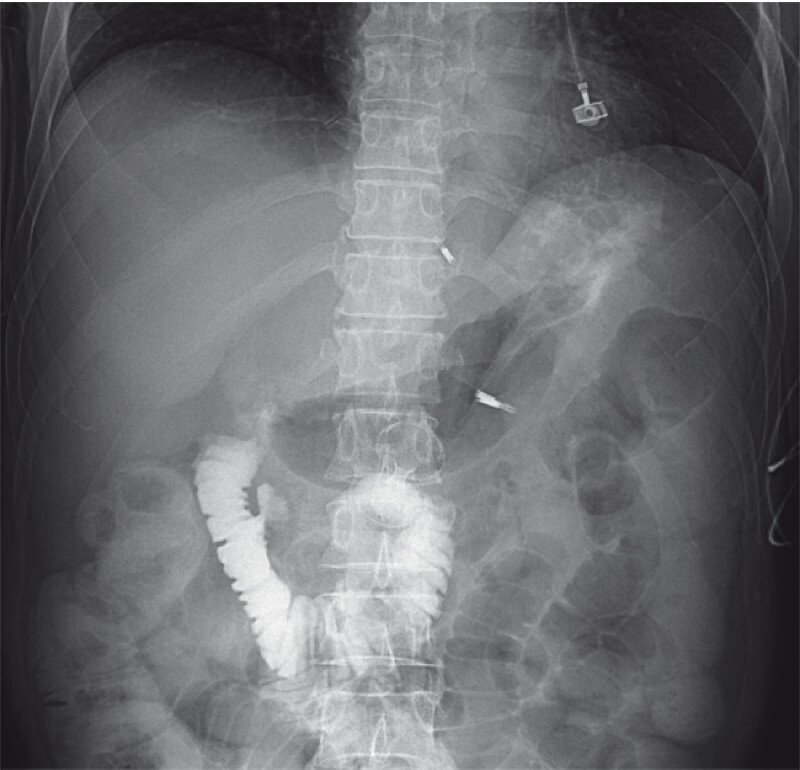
Radiographic image after a second endoscopic ultrasound-guided gastroenterostomy, using a new 20-mm cautery-enhanced lumen-apposing metal stent, had been successfully performed in the same session showing no obvious pneumoperitoneum.
